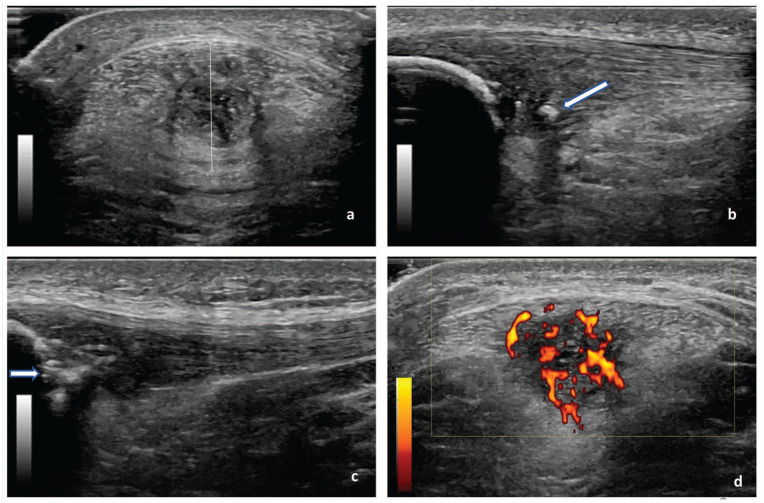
Patellar tendon assessed by US: (a) AP thickness measured in the transverse plane, (b) intratendinous calcifications (arrow) identified in the sagittal plane, and (c) patella erosions (arrow) identified in the sagittal plane. (d) PDUS: high level of Doppler flow (modified Ohberg score, 4) assessed in the transverse plane. These images are from different athletes with PT. AP, anteroposterior; PDUS, power Doppler US; PT, patellar tendinopathy; US, ultrasound.
